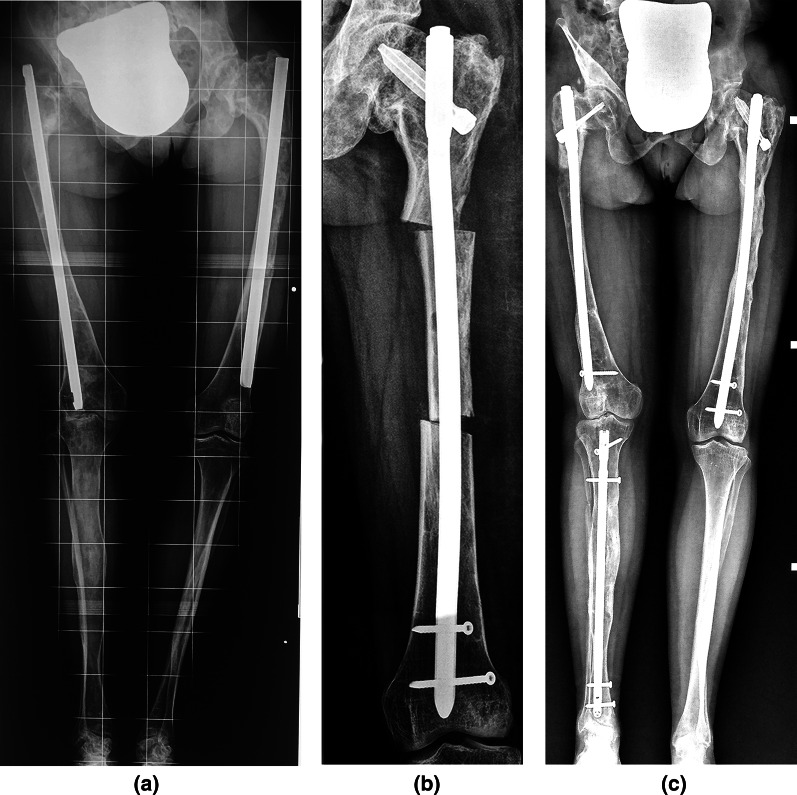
a Standing antero-posterior radiograph of the pelvis and lower limbs of a 22-year-old patient with McCune–Albright syndrome and bimelic fibrous dysplasia involvement, showing a bilateral shepherd’s crook deformity (type 6 femoral deformity), coxa brevis, medial bowing of the right tibia and 4 cm of lower limb length discrepancy. She had been operated on twice, with bilateral femoral Küntscher nailing performed at 10 and 17 years of age, respectively. She was able to walk with pain for only a short distance using 2 crutches, and her clinical Jung’s score was 2 points. b In our hospital, she had bilateral femoral and right tibial osteotomies stabilized with intramedullary nails. c At follow-up, 9 years later, she had 10° of residual varus deformity of the right femoral shaft, neck–shaft angles of 110° on the right and 125° on the left, 3 cm of knee height asymmetry, and 2 cm of lower limb length discrepancy compensated for by a shoe lift. She also had a slight bilateral limitation of the hip ROM, but she was able to walk independently for a long distance despite a mild painless limp. A good result was obtained, with a clinical Jung score of 8 points
